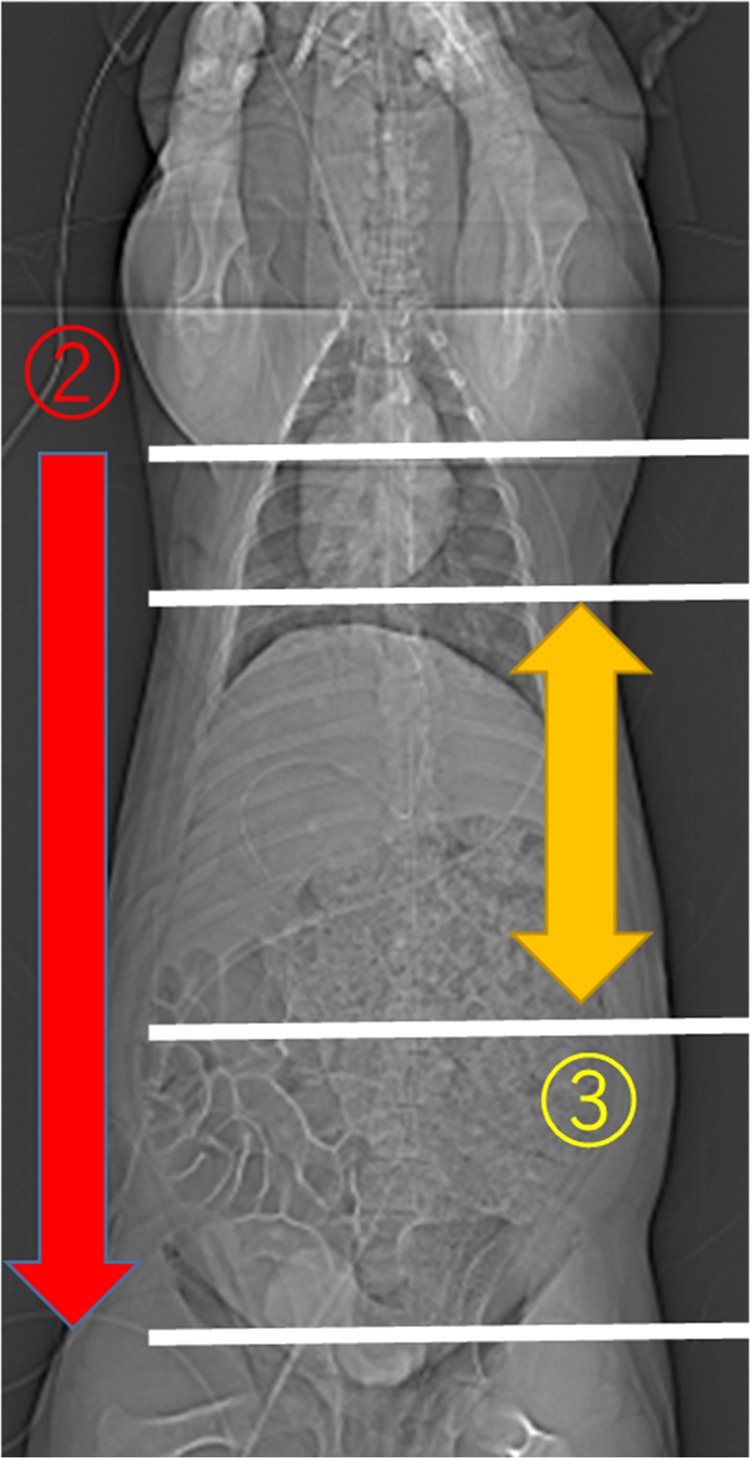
Scanning protocol (1) Scout view. Balloon positioning. (2) Pre-perfusion scan. Confirmation of the position of the inflated balloon. Non-enhanced, 3-mm slice. Scan range: from the supra-diaphragm to the entire abdomen. (3) Computed tomography perfusion. Blood flow and organ ischaemia. 3-mm slice Temporal resolution, 2 s + 4 s (multiple), 49 phases. Scan range: 204 mm from the top of the liver (69 slices). Tube potential, 120 kV; Tube current, 320 mA; Rotation time, 0.3 sec.
